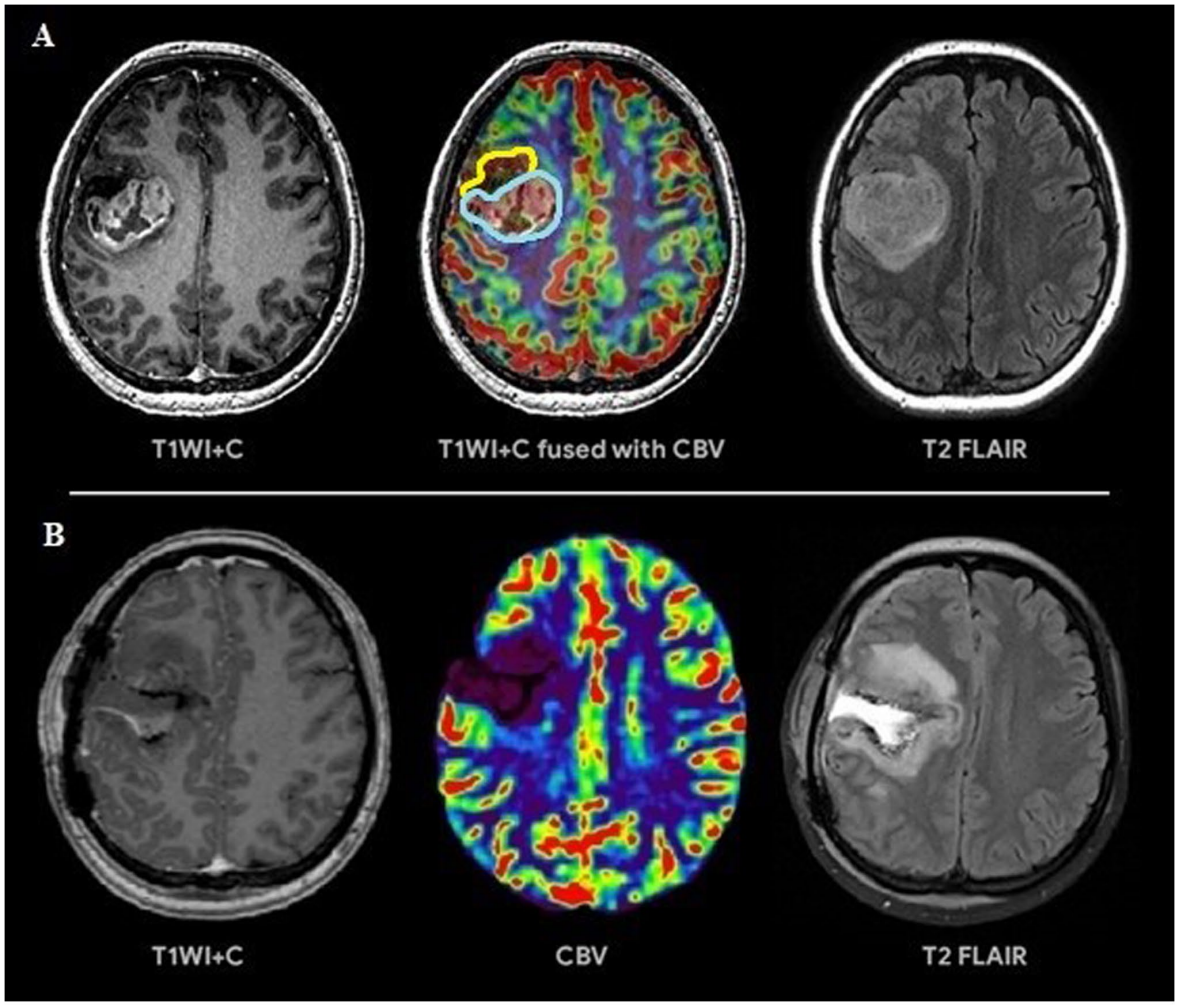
(A) Preoperative tumor resection planning in 42-years old patient with glioblastoma with regard to comparison of post-contrast T1 series, CBV parameter obtained using DSC-T2* perfusion and T2-Flair. The tumor has 2 portions: contrast-accumulating (blue line) and contrast-negative with high CBV volume (yellow line), which correspond to each other. (B) Postoperative MRI control 24 h after operation, post-contrast T1 series, CBV indicator obtained during DSC-T2* perfusion and T2-Flair. MRI data reveal resection of the contrast-accumulating component and the component with high CBV values and non-accumulating contrast agent; a zone of perifocal edema-infiltration is visualized in T2-Flair.
